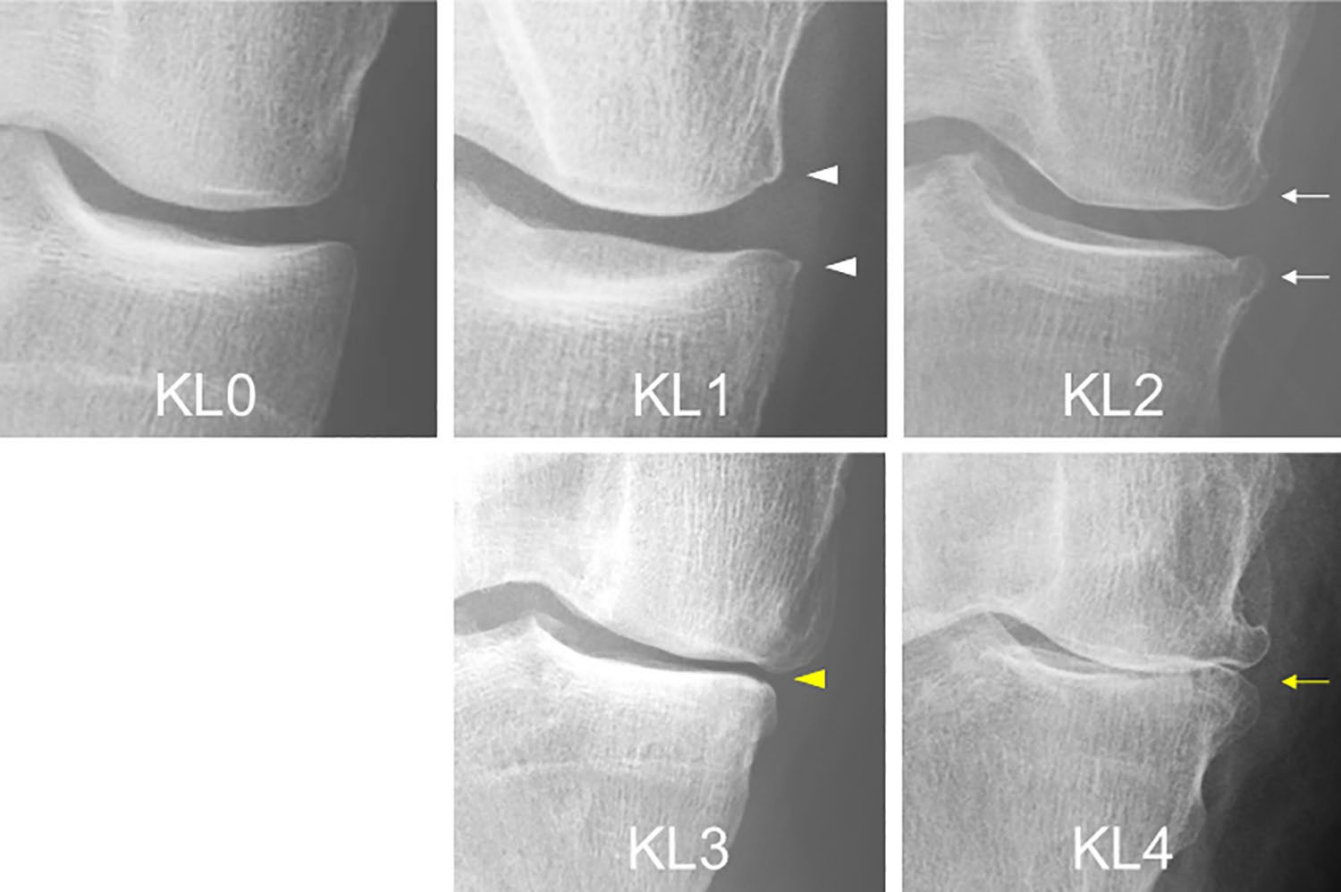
Representative radiographs of KL grading in medial OA. KL grade 0 shows no features of OA. KL grade 1 shows osteophytic lipping (equivocal osteophytes) (white arrow heads). KL grade 2 shows definite (unequivocal) osteophytes (white arrows). KL grade 3 shows joint space narrowing (yellow arrow head). KL grade 4 shows definite deformity of the bone ends (bone-to-bone appearance) (yellow arrow).
